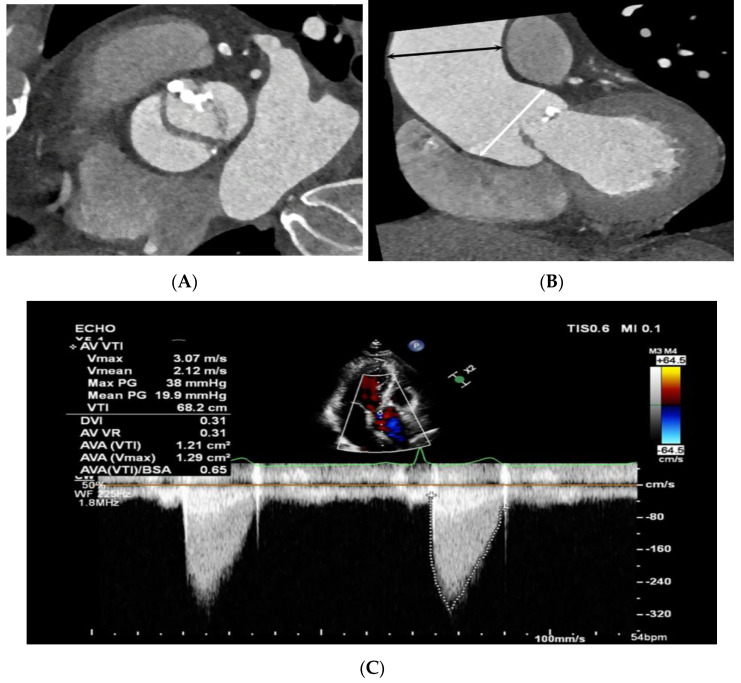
Bicuspid aortic valve (BAV) without a raphe and combined aortic root, as well as mid-ascending aorta in a 64-year-old male. (A) Double-oblique CT reconstruction parallel to the aortic valve demonstrates thickened, calcified BAV cusps without a raphe. (B) Diameters of the sinuses of Valsalva (white arrow) at 43.5 mm (size index 2.24 cm/m^2^) and the mid-ascending aorta (black arrow) at 46.4 mm (size index 2.48 cm/m^2^), which were measured at mid-systole. (C) Continuous-wave Doppler recording of the aortic stenosis jet from an apical approach shows a maximum velocity of 3.07 m/sec. The continuity equation of the aortic valve area was 1.2 cm^2^, corresponding to moderate aortic stenosis.
